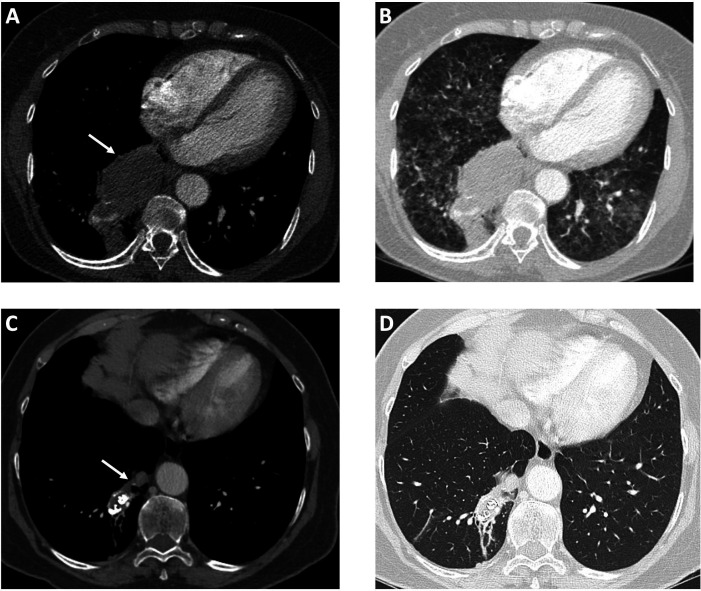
The figure shows a CTA performed at admission, before endovascular treatment (A) soft tissue window and (B) lung window; and a follow-up CTA performed 56 months after the intervention (C) soft tissue window and (D) lung window. A significant decrease in pulmonary sequestration size is observed, from 77 × 40 mm in 2016 to 28 × 18 mm in 2021 (white arrows in A and C).
